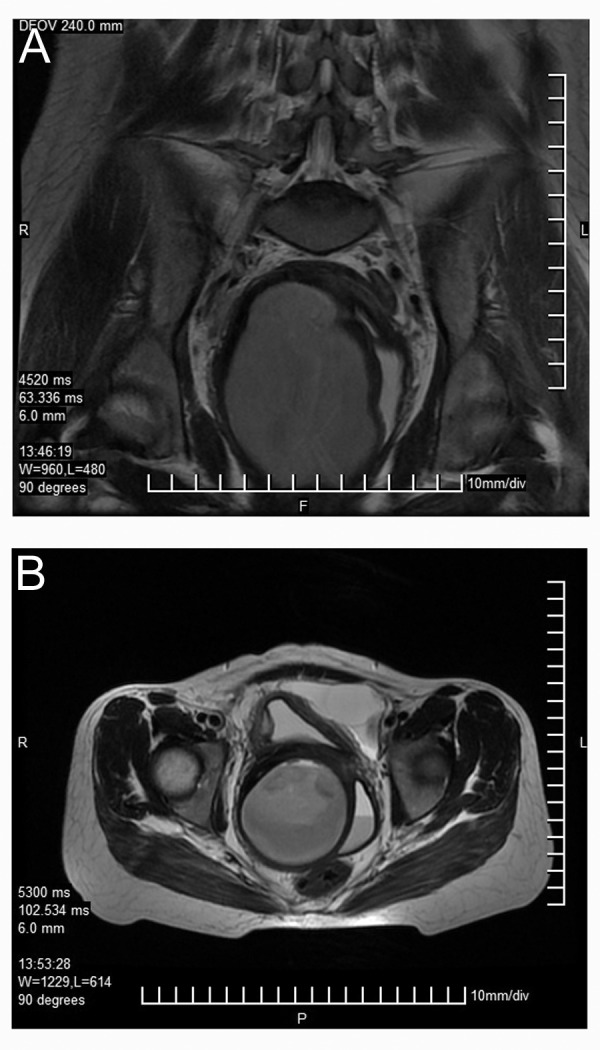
A. Coronal and B. Axia T2W MRI images showing distended
